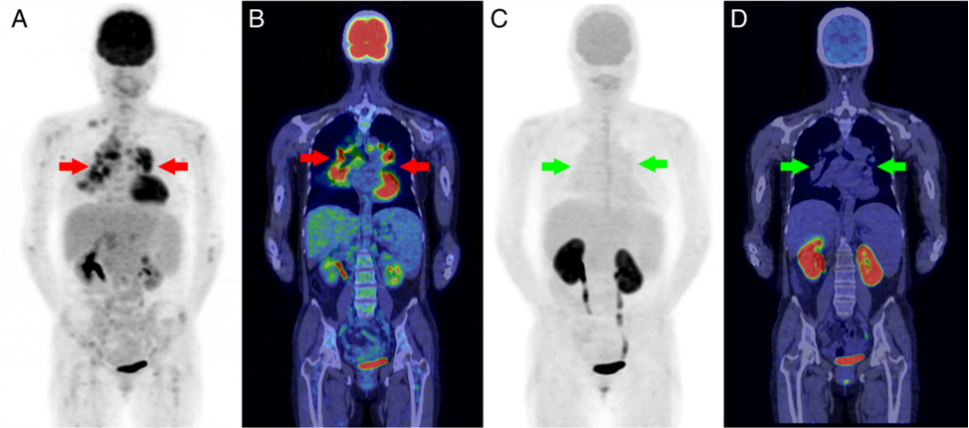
PET/CT images in a 54-year-old female with sarcoidosis–lymphoma syndrome after chemotherapy. Whole-body scan of FAMT PET/CT was performed at 60 min after 281.7 MBq of FAMT injection. (a-b FDG PET/CT; c-d. FAMT PET/CT). High FDG uptake in the mediastinal lymphadenopathies (*red arrow*) was still seen. The FDG–avid liver tumor lesions disappeared. No FAMT uptake that was compatible with sarcoidosis lesions was seen in the mediastinal lymphadenopathies (*green arrow*). *FDG*
